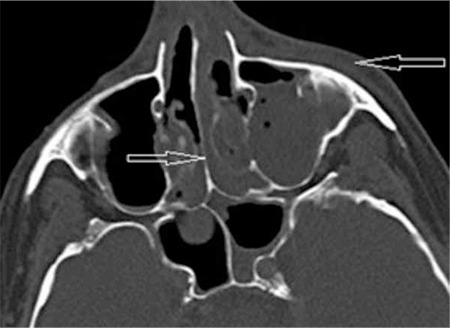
Paranasal sinus CT scan revealed a defect of the nasal septum and skin and subcutaneous edema.
